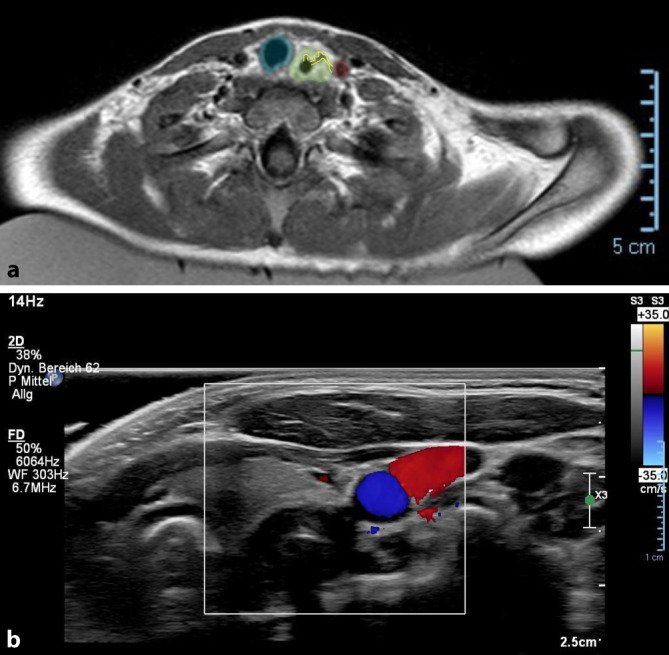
a MRT präoperativ. Trachea (*blau*), ödematöser Ösophagus (*grün*) mit 2 gangbildenden Ulzerationen (*gelb*), von denen einer bis in die Nähe der ACC (*rot*) reicht. b Farbkodierte Duplexsonographie: Ösophaguswand ohne erkennbare trennende Strukturen zur ACC (Farbduplex, *blau*)
